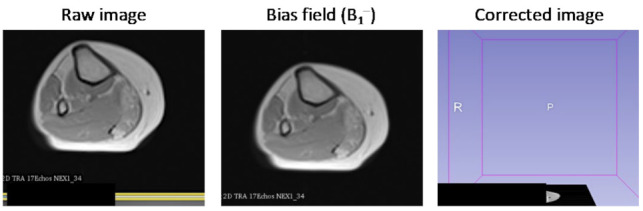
Correcting receiver gain bias in an axial MRI of the calf. Left: Image affected by inhomogeneous B_1_^–^ intensity bias; Middle: bias field, estimated using the N4ITK method; Right: image after intensity correction.
